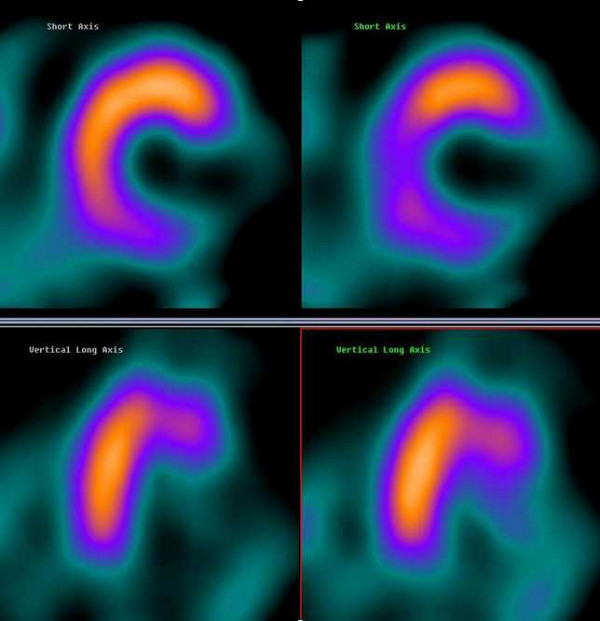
Post-nitrate myocardial (left images) and rest (right images) ^99m^Tc-tetrofosmin SPECT showing absent uptake of tracer in lateral and infero-lateral wall. The nitrate administration did not significantly change the perfusion pattern.
